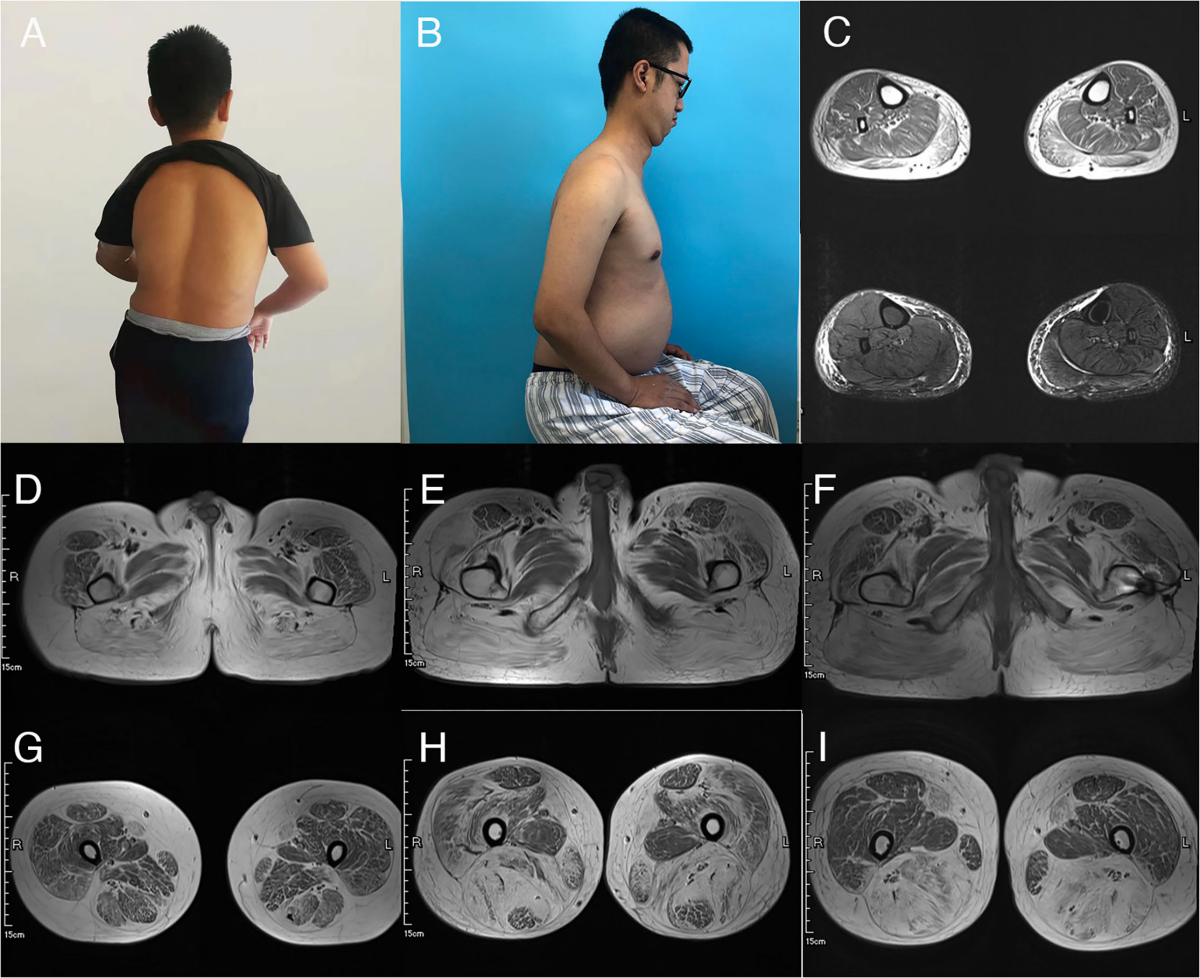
MRI findings of the thigh and photographs of patients. Scoliosis in patient one and rigid spine in patient two were showed in (A,B). (C) Showed adipose infiltration of bilateral gastrocnemius in patient three in T2-weighted image (T2WI) (upper) and fat-saturated T2-weighted sequence (lower). Abnormal signals in bilateral gluteus maximus and subcutaneous adipose accumulation were revealed in patient one (D), patient two (E), and patient three (F). MRI of the thigh muscle of patient 1 showed overall thigh muscle mass atrophy and diffused abnormal signal. Bilateral sartorius was the most severely involved and almost replaced by adipose tissue (G). Patient two showed massive involvement of thigh muscles (H) with overall fat infiltration, except the rectus femoris and adductor longus. The quadriceps involvement was severe and asymmetrical, left more than right. Thigh muscles from patient three showed severe fat infiltration particularly in the posterior compartment and bilateral sartorius. Other muscles were relatively preserved (I).
